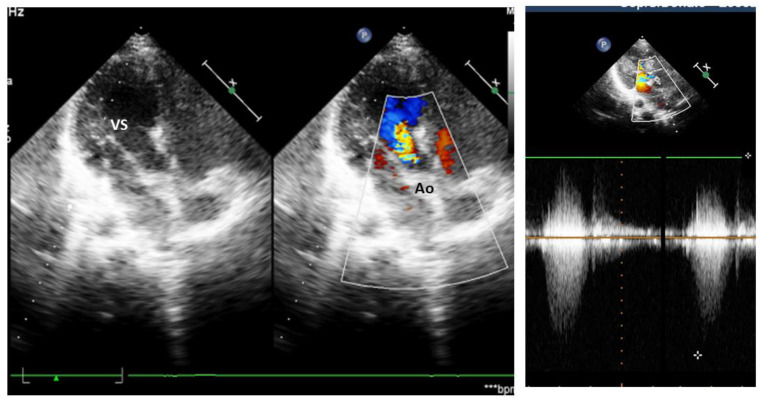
Echocardiography at 9 yrs. Only a small echodensity of a residual tumor is evident in the interventricular septum, with turbulent flow toward aorta, evident at color Doppler and with increased velocity at continuous wave Doppler at the right panel. (VS—left ventricle, ao—aorta).
